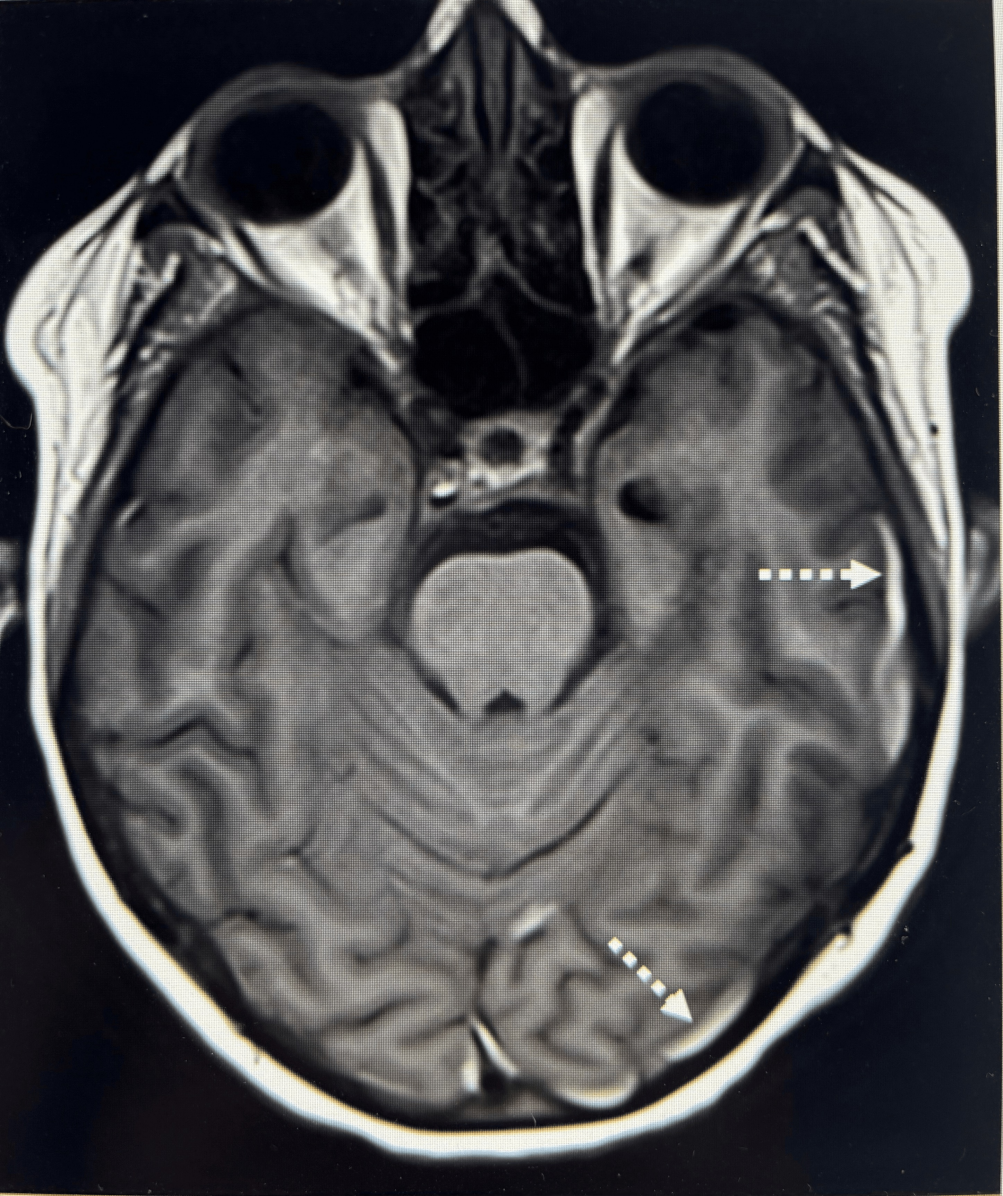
Brain MRI (Patient 2) Brain MRI of Patient 2, performed before EBP. Axial unenhanced T1-weighted sequence reveals subdural hemorrhage shown by thin, hyperintense subdural fluid collections in the left temporal and occipital regions (dashed arrows). EBP: epidural blood patch
